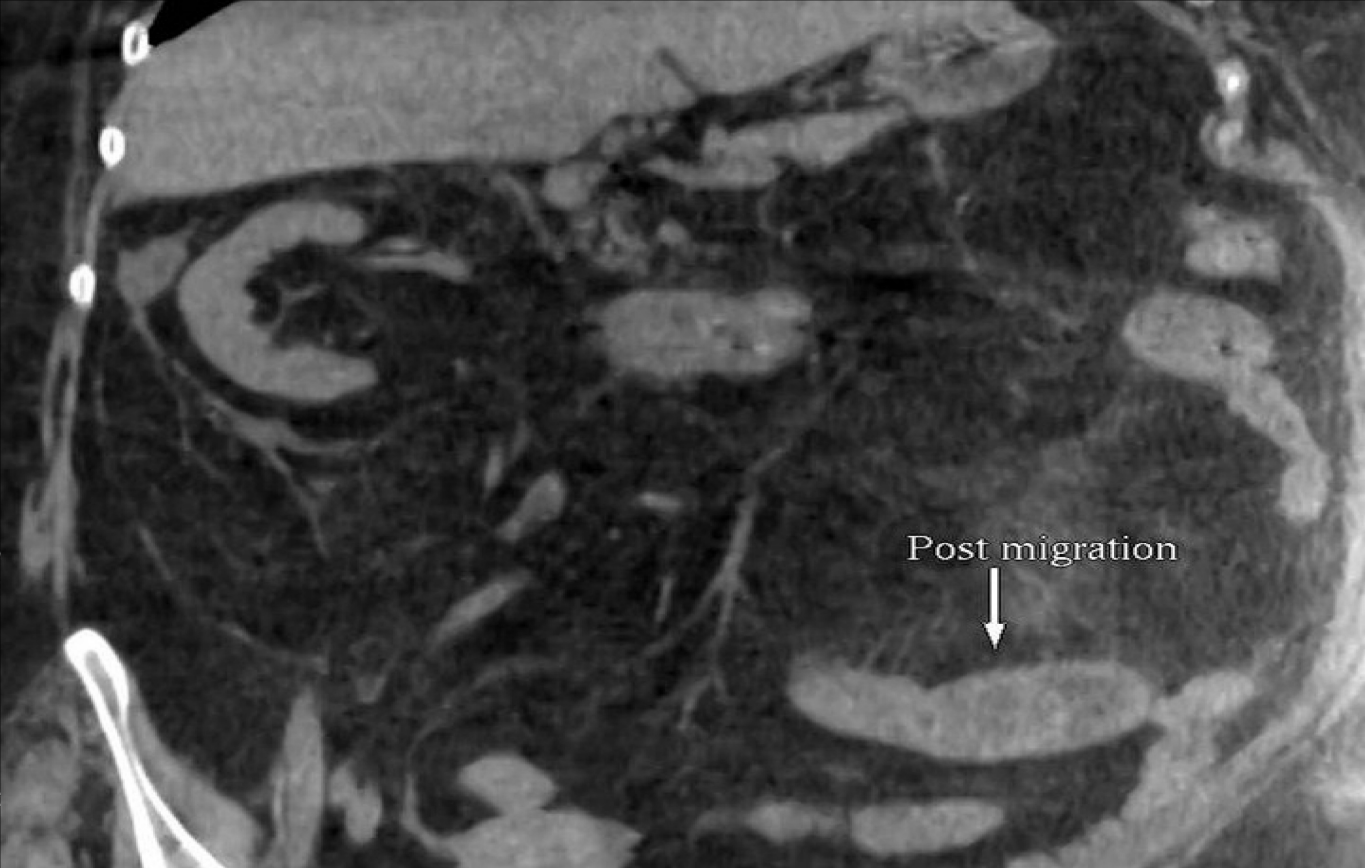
Abdominopelvic CT scan coronal cut showing the migration of the obstructing stone to the jejunum (arrow) CT: computed tomography
